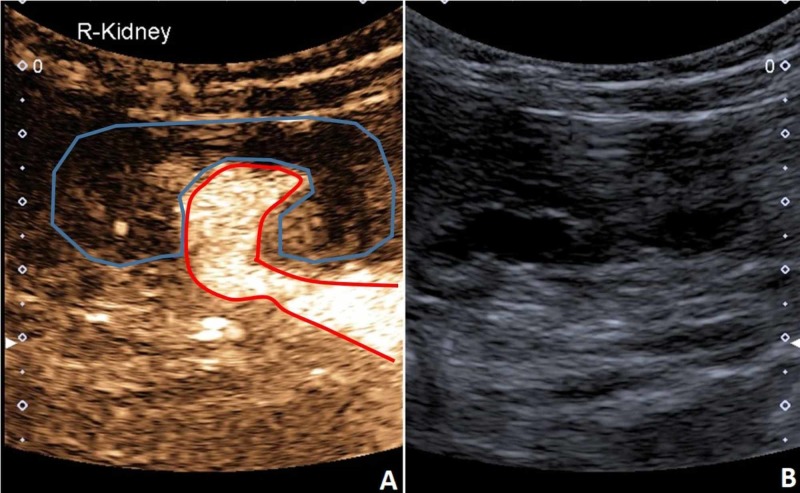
CEUS (A) demonstrating retrograde filling of the lower part of the duplicated kidney collecting system (red outline). Rough margins of the kidney (blue outline). (B) B-mode US of the kidney. CEUS, contrast-enhanced ultrasound US, ultrasound
